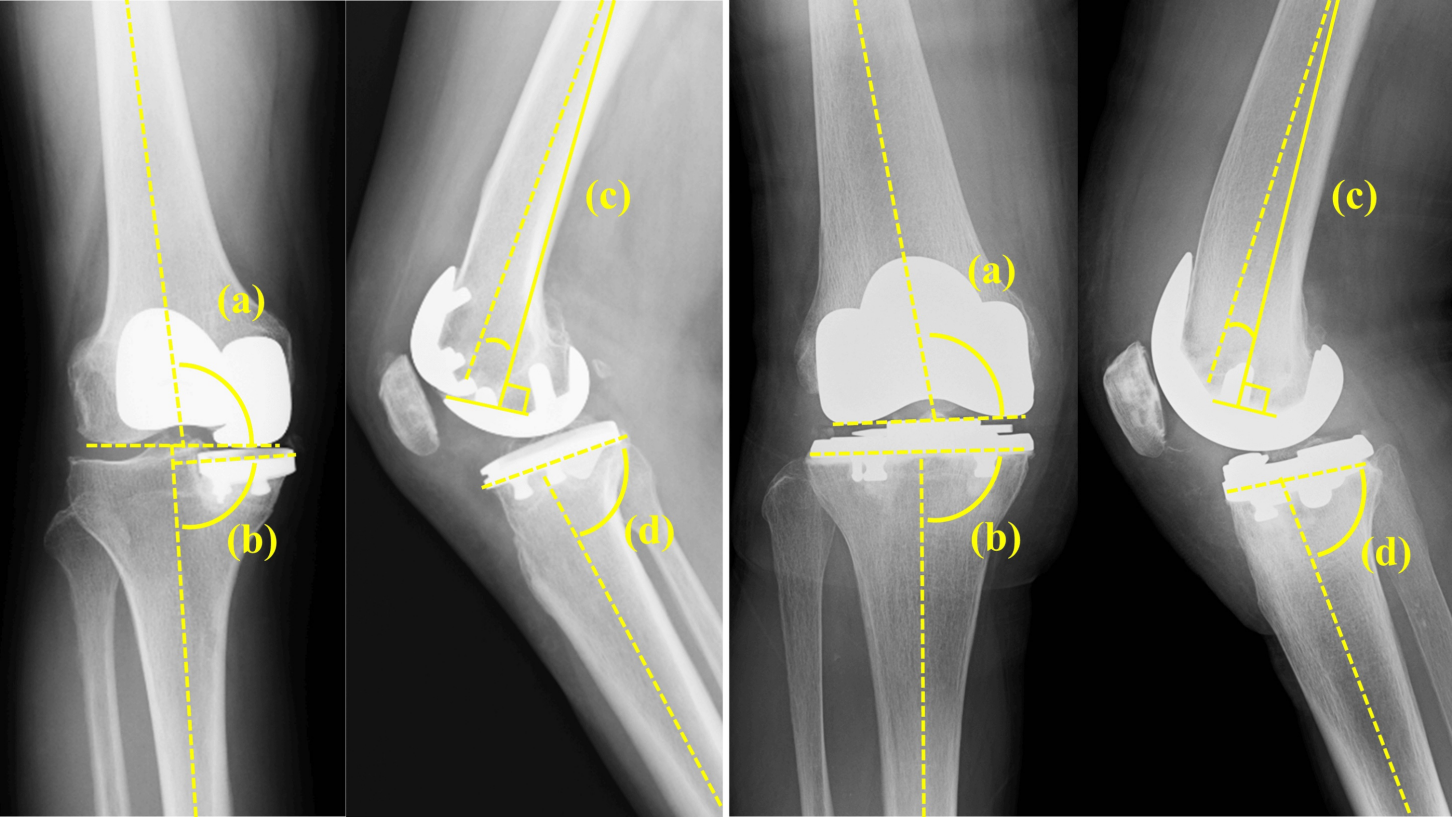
Postoperative implant installation angles. Coronal alignment is evaluated on the AP radiograph. The coronal alignment of the femoral component (a) is defined as the angle between the distal femoral component surface and the anatomic axis of the femoral shaft. The tibial component coronal alignment (b) is determined as the angle between the baseplate and the mechanical axis of the tibia. Sagittal alignment is evaluated on the lateral radiograph. The femoral component flexion angle (c) was measured as the angle between the most distal femoral fixation surface and the femoral shaft axis, with an anterior angle defined as positive. The tibial component sagittal angle (d) is determined as the angle between the baseplate and the anatomical tibial axis.
